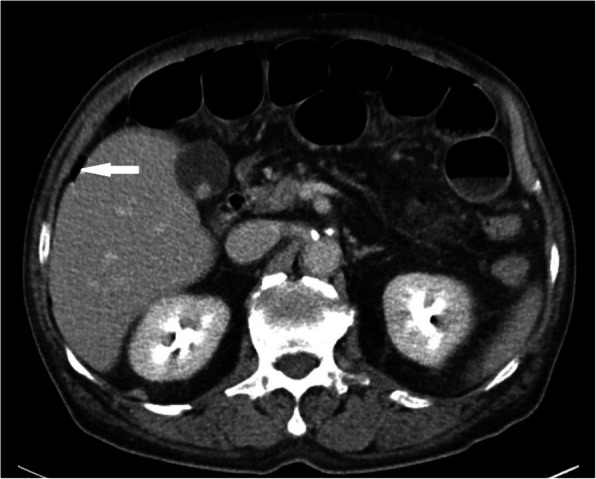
Abdominal computed tomographic findings. Free air in liver surface (white arrow) were observed
